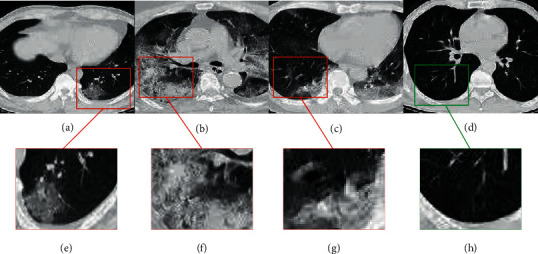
Chest CT images of laboratory-confirmed COVID-19 patients and 1 healthy subject. Patients' GGOs are highlighted with red borders, and normal subjects' GGOs are highlighted with green borders. (a–c) Lung CT images of COVID-19 patients in the early, advanced, and absorptive stages, respectively; (e–g) the parts of GGO in these three stages, respectively; (d) a CT scan of a normal lung; (h) a local magnification of a normal lung.
